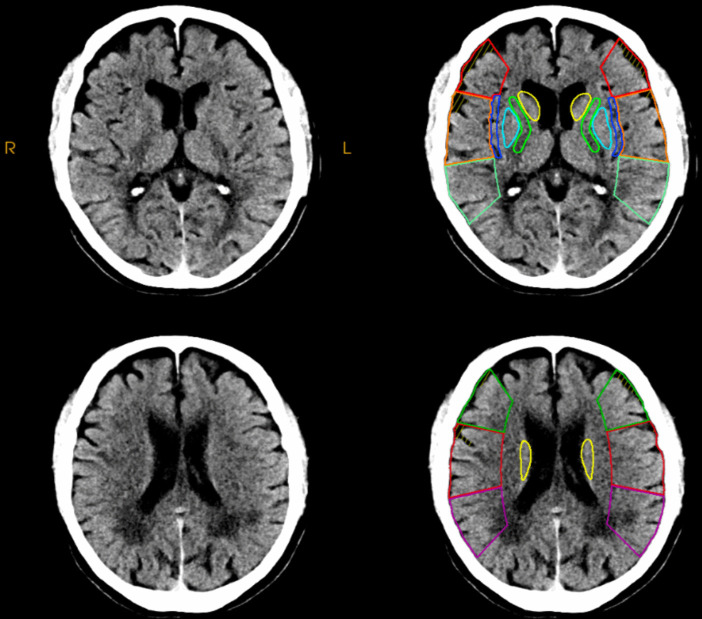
Non-contrast head computed tomography (CT) depicting the Alberta Stroke Program Early CT Score (ASPECTS)
